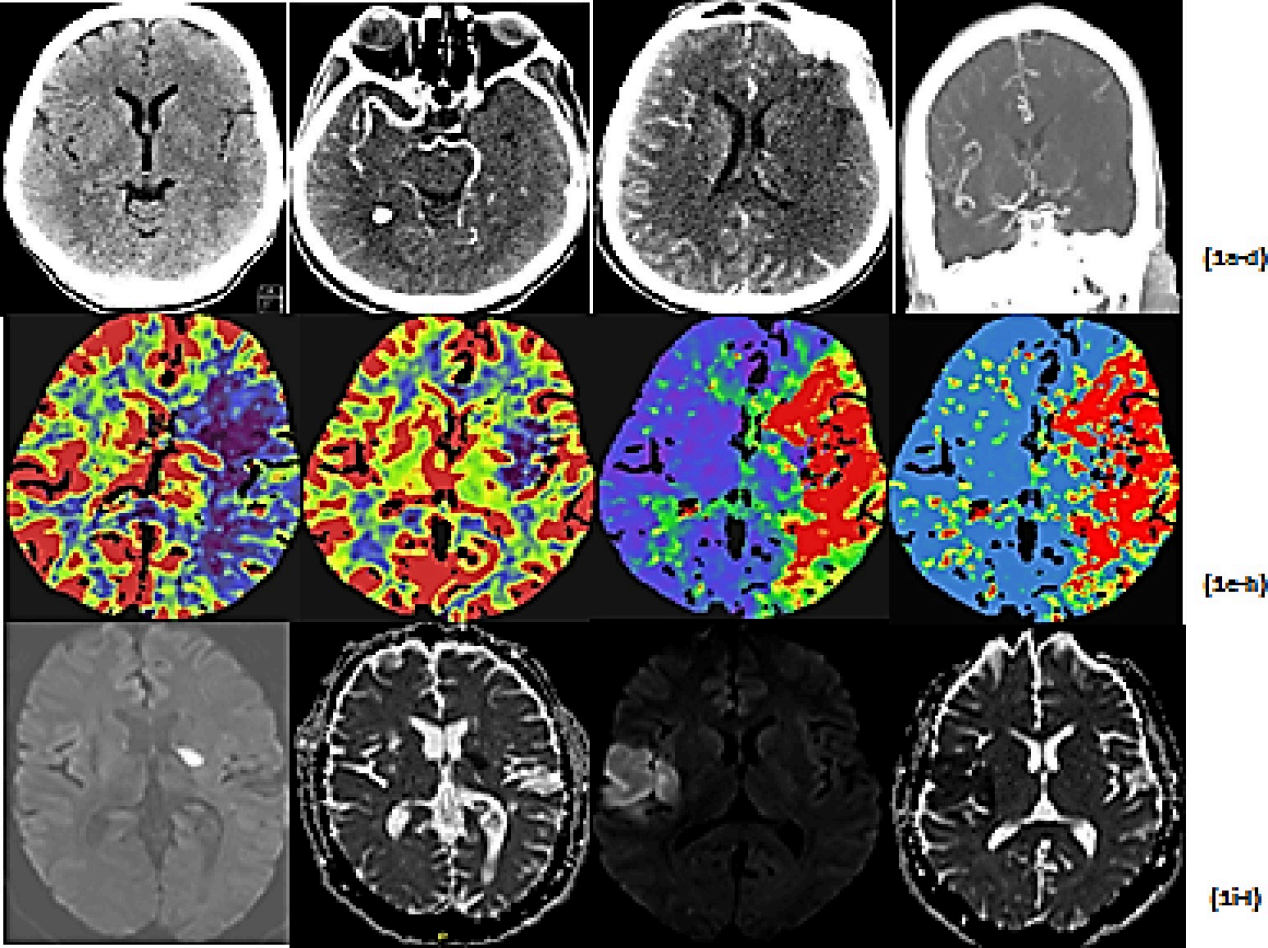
Localisation of stroke type based on imaging, (a) showing non-contrast CT brain, (b) CT angiogram showing occluded left Middle cerebral artery, (c) and (d) paucity of collateral circulation left MCA vessel, with (e) showing reduced cerebral blood flow in defined territory, (f) cerebral blood volume, (g) increased time to drain, and (h) increased mean transit time, (i) and (j) showing DWI and ADC map showing Lacunar stroke, and (k) and (l) showing DWI and ADC scan of a Cortical Stroke.
